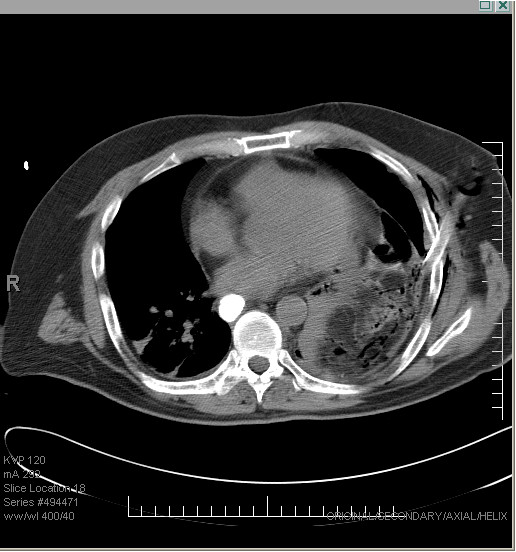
Abdominal CT scan after placement of chest tube. Reveals presence of loops of bowel in thoracic cavity as well as fluid in left hemithorax. Note also mediastinal shift to the right side.
